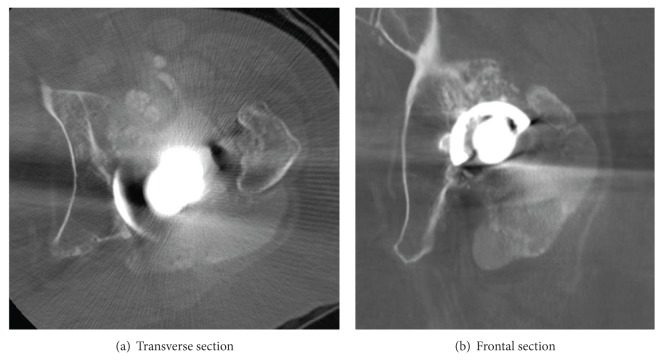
CT findings. Multiple calcified lesions and accumulated fluid in the capsule and in bursa were observed.
